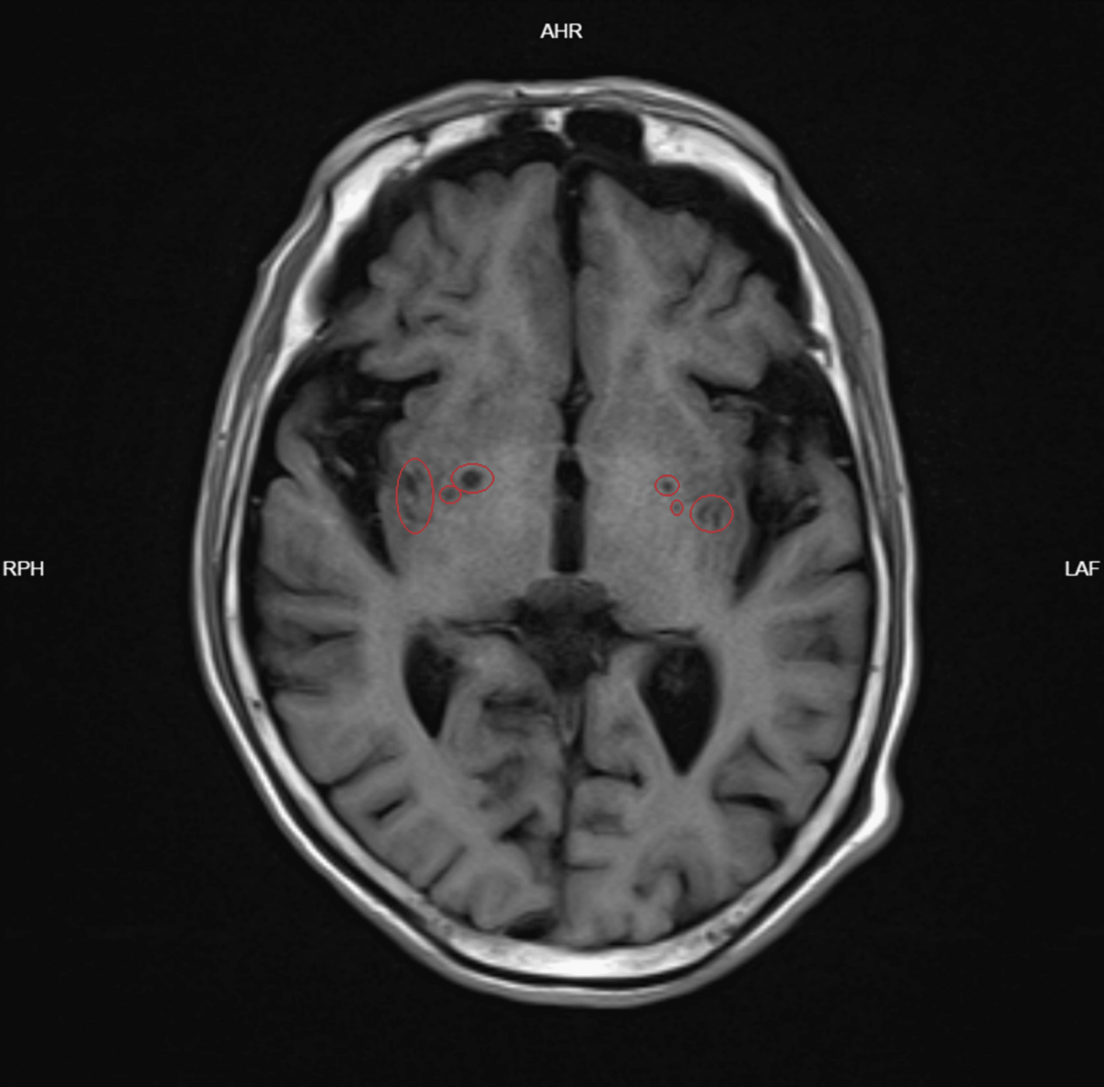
MRI T1WI showing bilateral basal ganglia lacunar infarcts The red circles show chronic small-vessel disease. T2WI - T2-weighted imaging
